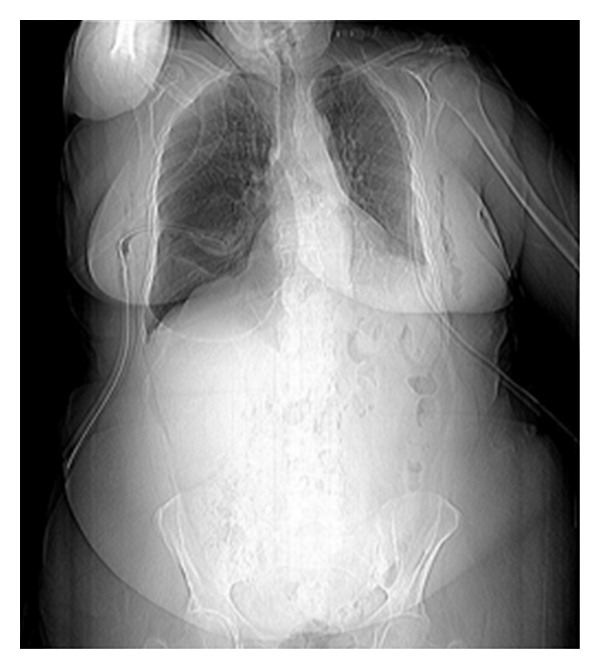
A CT Scanogram showing bilateral chest drains the right drain is inappropriately placed in a right basal bulla.
